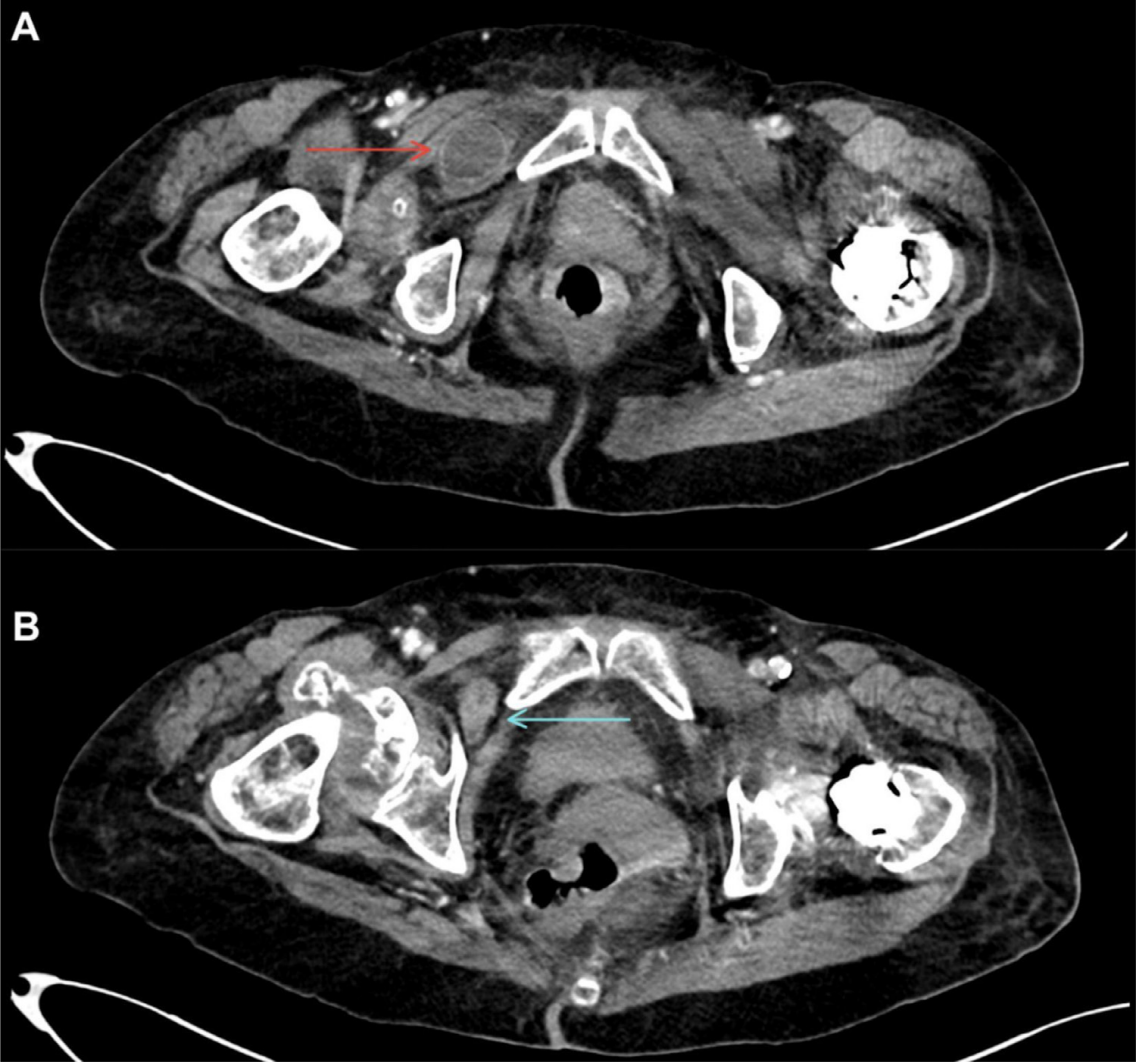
Contrast-enhanced abdominal computed tomography images in the portal venous phase, axial acquisition. (A) An ileal loop (red arrow) is identified between the fibers of the right external obturator muscle, deep to the right pectineus muscle. The loop shows no intraluminal contrast, has normal wall thickness, and demonstrates homogeneous enhancement. There is no evidence of hyperdense or loculated fluid within the hernia sac or adjacent mesenteric folds, nor is there intramural gas. Additionally, no portal venous gas or pneumoperitoneum is identified elsewhere in the study. (B) On a more cranial slice, the herniated loop is visualized passing through the right obturator foramen, appearing collapsed and creating a clear transition zone (blue arrow).
